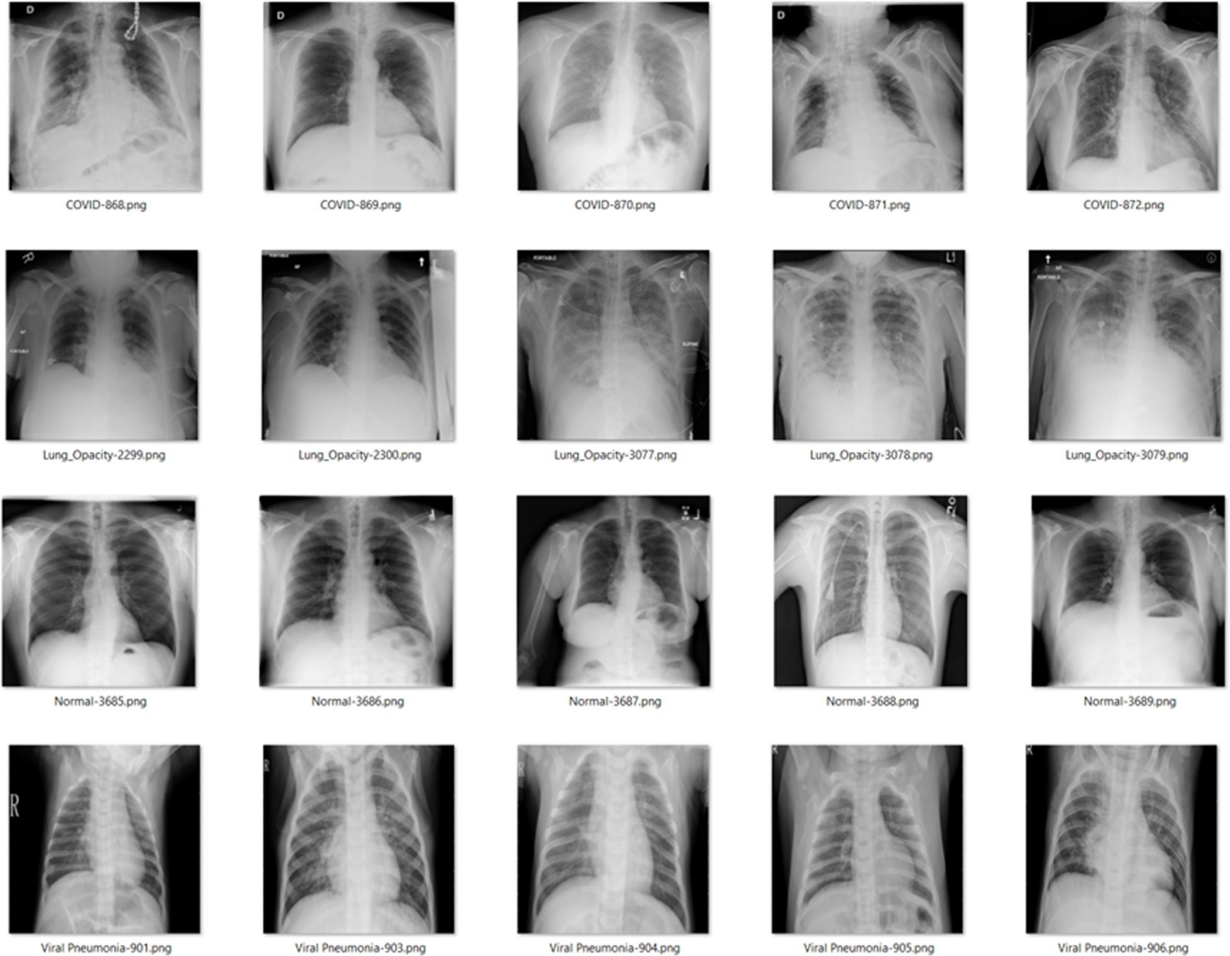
Radiography X-ray image from the dataset.
